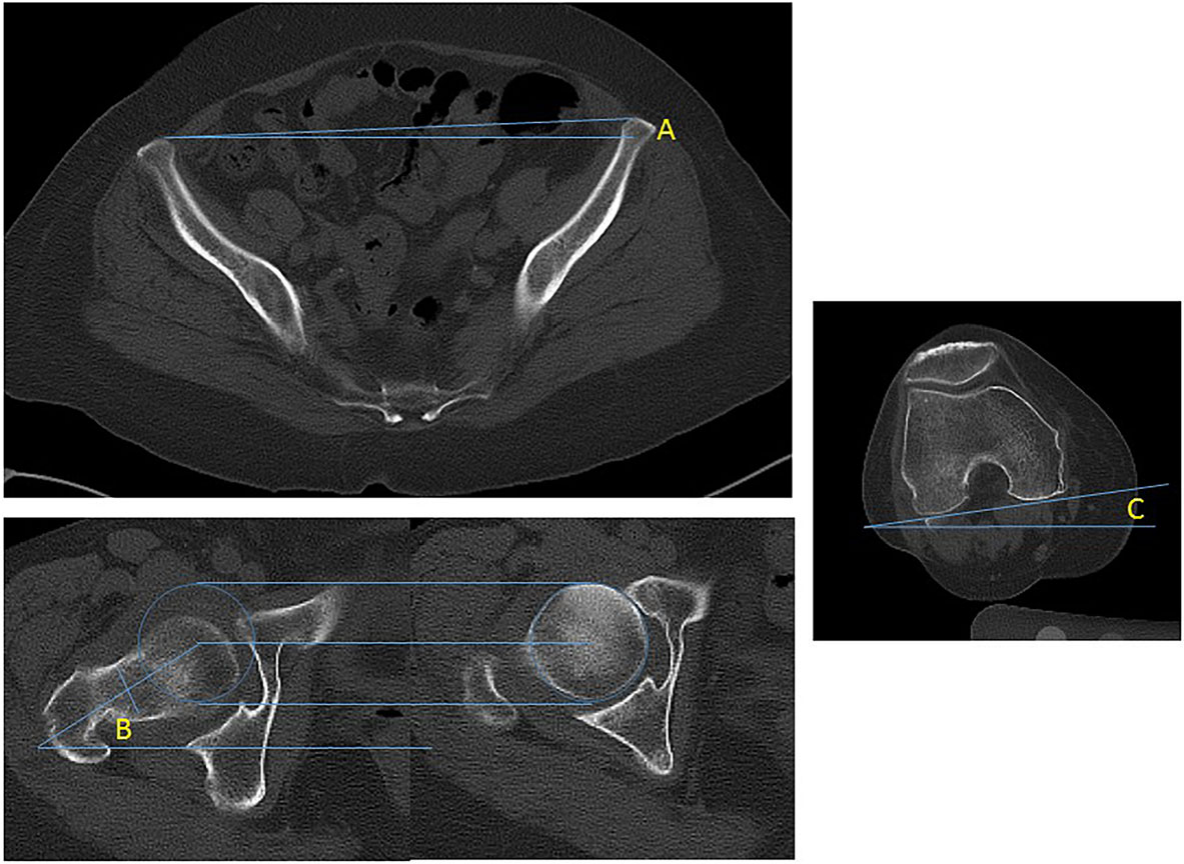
Measurements on pelvic and lower extremity axial computer tomography (CT). Angle a is defined as the angle between the line of the CT berths and the line connecting both anterior superior iliac spines. Angle b is defined as the angle between the line of the CT berths and the line connecting the femoral head center with the neck center. Angel c is defined as the angle between the line of the CT berths and the posterior condylar line
